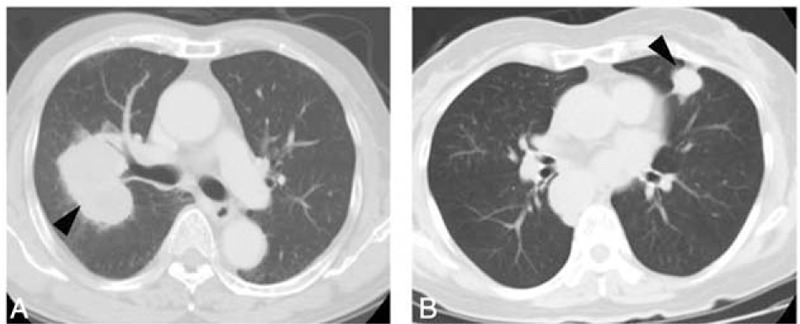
Typical thin-sliced computed tomography (CT) pictures of central (a) and peripheral (b) types of small cell lung carcinoma (SCLC). Each arrow indicates the primary mass. For definition of central and peripheral tumors, see the text. CT = computed tomography, SCLC =  small cell lung carcinoma.
